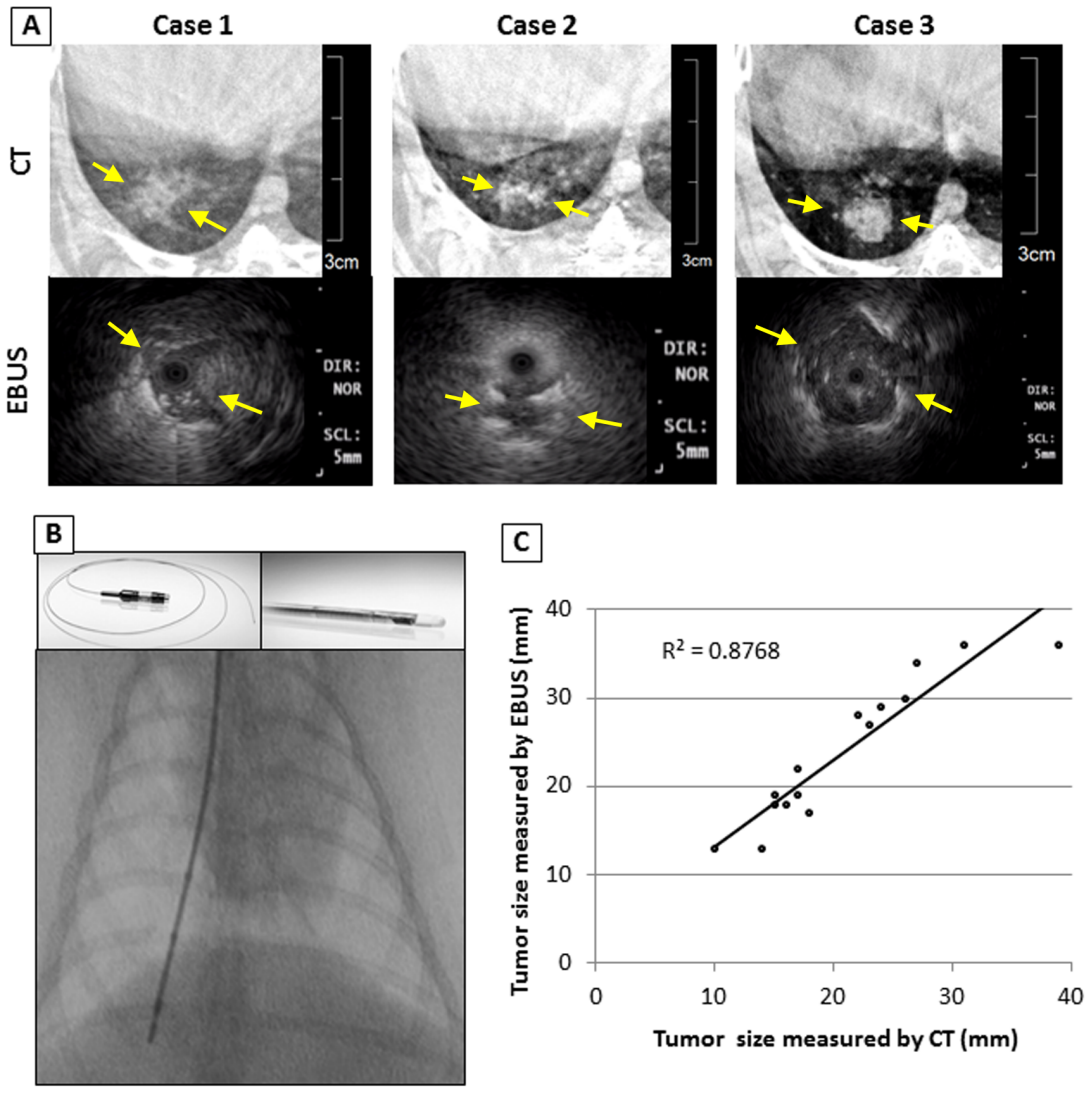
Comparison of CT and EBUS images. A: CT and EBUS images of the 3 representative cases. EBUS visualized axial images of the lung tumor. The tumor was characterized as the iso-echoic heterogeneous mass lesion with high echoic margin. B: EBUS was led to the lung tumor under fluoroscopy guidance. C: EBUS measurement of tumor size was strongly correlated with that of the CT ((n = 16, R^2^ = 0.8768; R^2^: the Pearson Coefficient of Determination).
